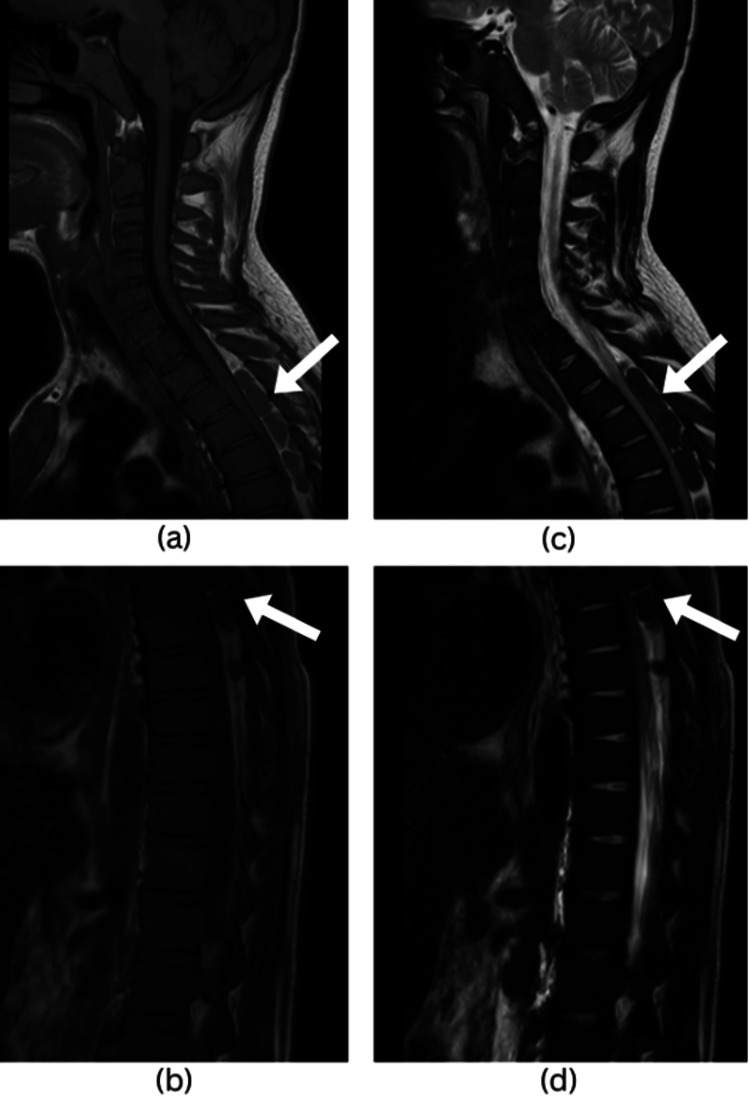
Pre-op MRI of the dorsal spine. Sagittal T1-weighted images (a,b) and sagittal T2-weighted images (c,d) show an epidural isointense lesion (marked by arrows), located posteriorly and compressing the spinal cord from T3 to T8.
